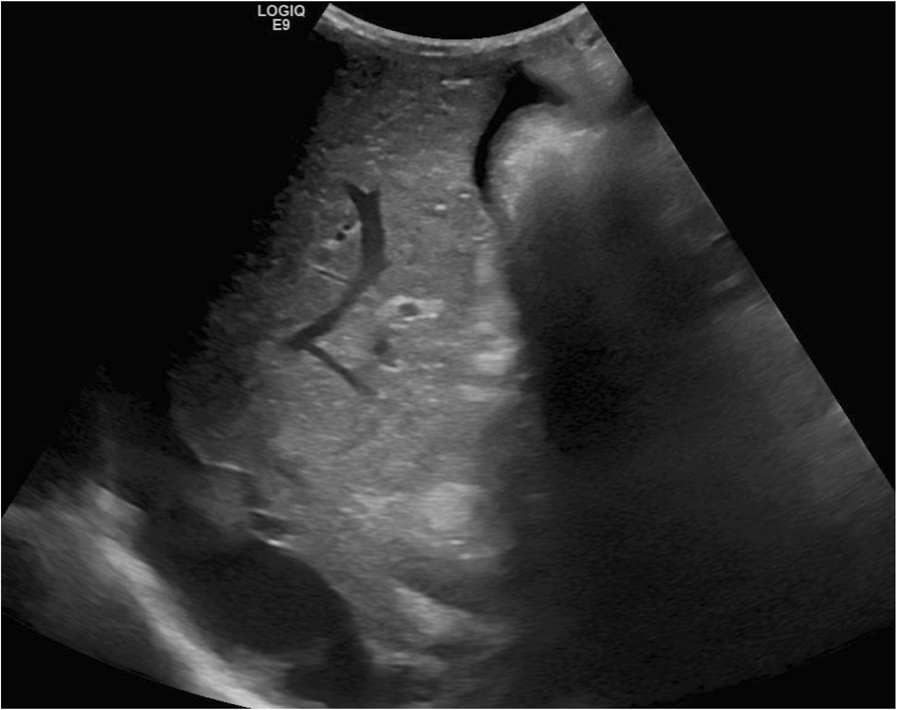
Liver cirrhosis in pediatric Gaucher disease. Sagittal grayscale ultrasound demonstrates an enlarged liver with nodular cirrhotic morphology and perihepatic ascites in a 12-year-old male with Gaucher disease
